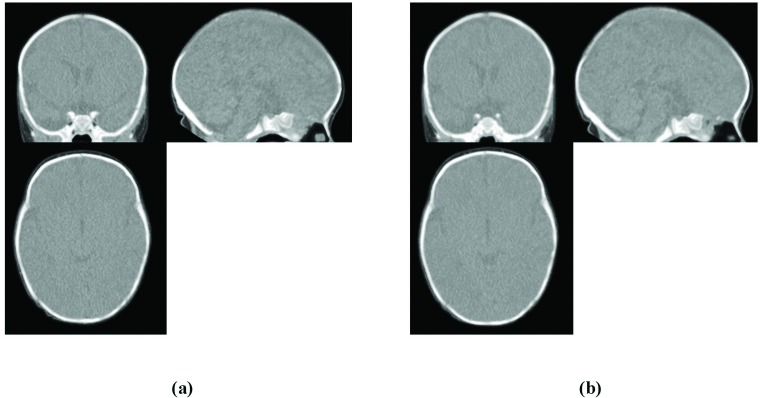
CT'-MR co-registration, second step: whole head intensity-based registration. (a) CT' image obtained by applying the resulting deformation field from CT-MR intracranial co-registration sub-section to the preprocessed CT image. (b) CT'' image achieved by linearly registering CT' to GRAMFC_T_39-42_.
